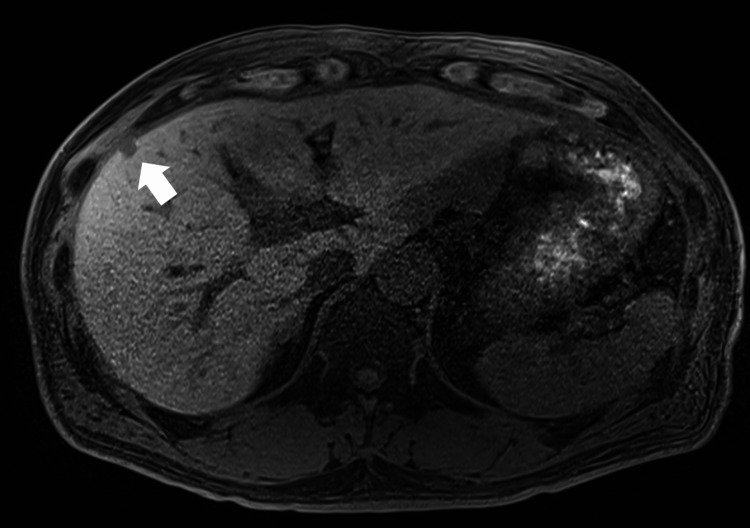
Magnetic resonance imaging T1 hepatobiliary phase of Case 1 revealed a small low-intensity mass (arrow) on the surface layer in segment 5 of the liver.
